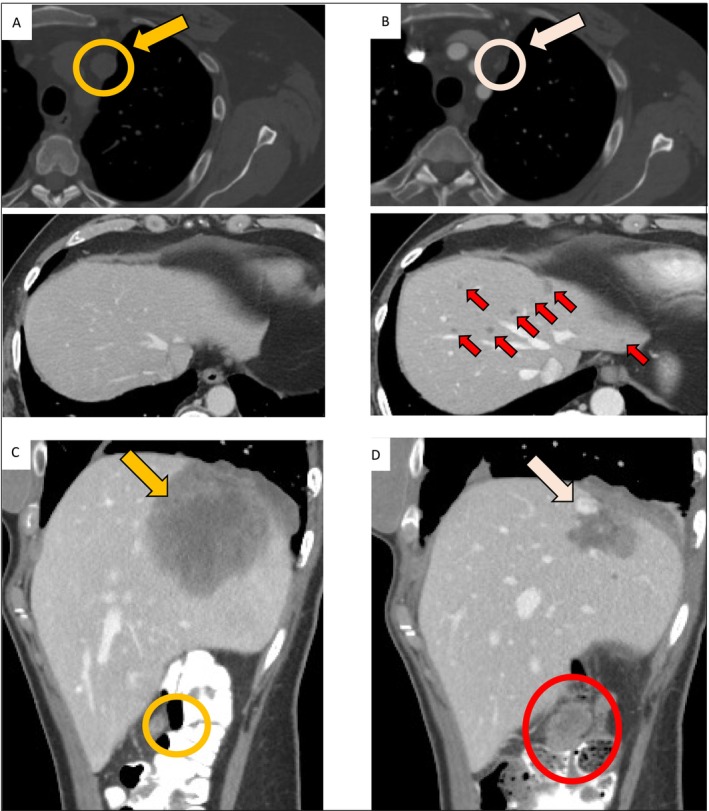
Representative images of mixed responses to NOTCH inhibitor. (A, B) Computerized tomography (CT) of the chest (top) and abdomen (bottom) at baseline (A; yellow circle and arrow) and at the second re‐staging at 16 weeks (B) demonstrates response in the mediastinal nodal metastasis (white circles and arrows) but development of new liver metastases (red arrows). (C, D) CT of the abdomen in another NOTCH inhibitor trial patient at baseline (C; yellow circle and arrow) and at second re‐staging at 16 weeks (D) demonstrates response in a hepatic lesion (white arrows), but progression of a peritoneal metastasis (red circles).
